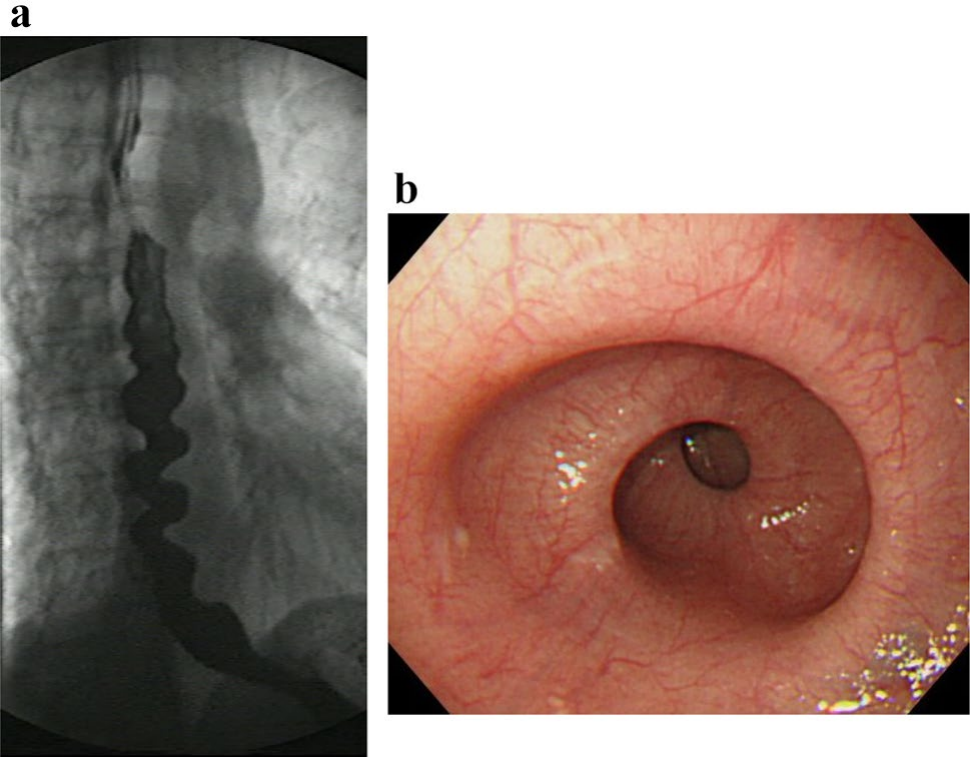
X-ray (a) and endoscopic (b) images of nutcracker esophagus
